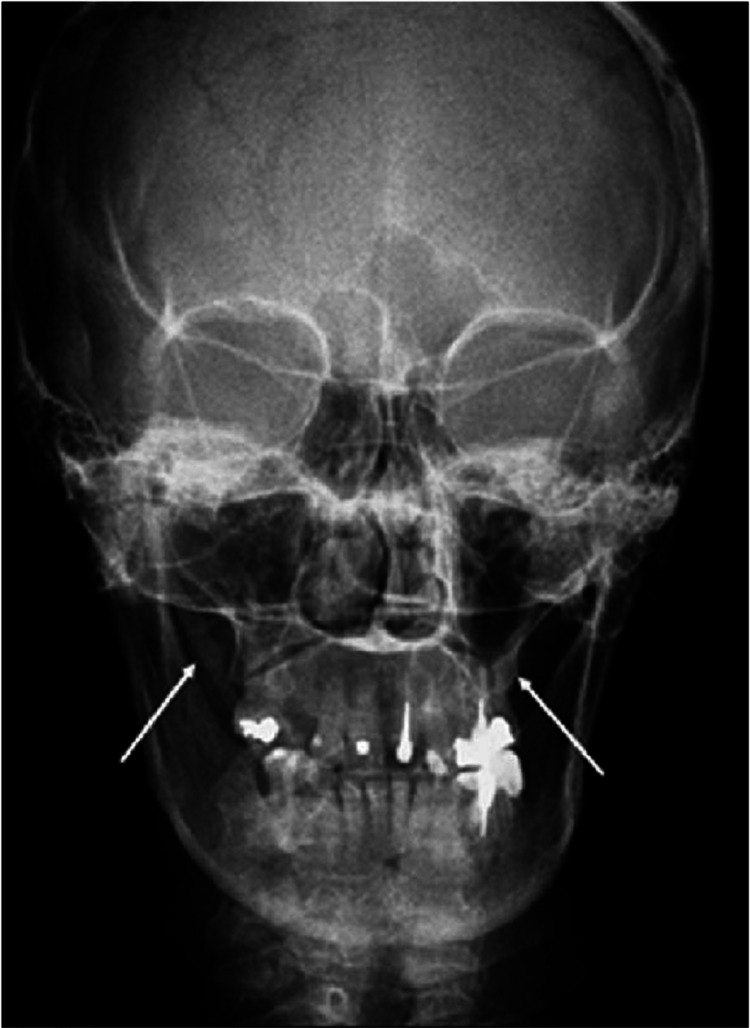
X-ray: reverse Towne’s view showing the elongated styloid process of the patient denoted by white arrows.
